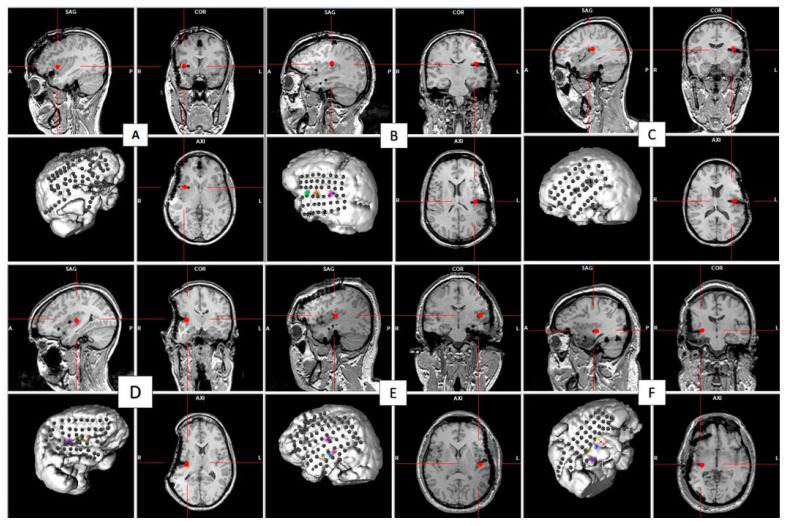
The 3-D representations of each patient’s brain with icEEG recording sites and location of the insular site of stimulation (small red cross) on the sagittal, axial, and coronal T1-weighted MRI cuts. The stimulation site was in the anterior insula in patient 1 (A) and in the posterior insula in the others ((B–F) was for patients 2, 3, 4, 5 and 6 respectively).
